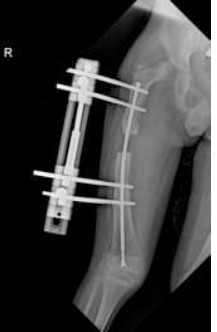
anteroposterior hip X-ray—excentration of the femoral head following distraction
